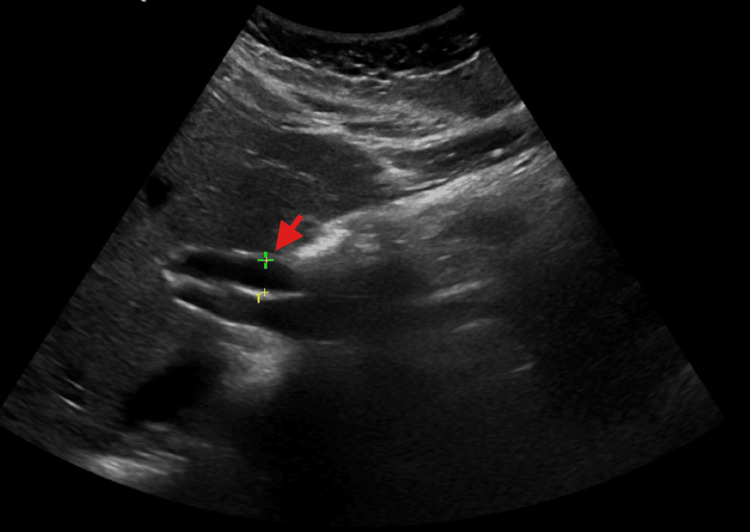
Ultrasound image of abdomen demonstrating dilated common bile duct (red arrow) of 7.2 mm (case 2).
